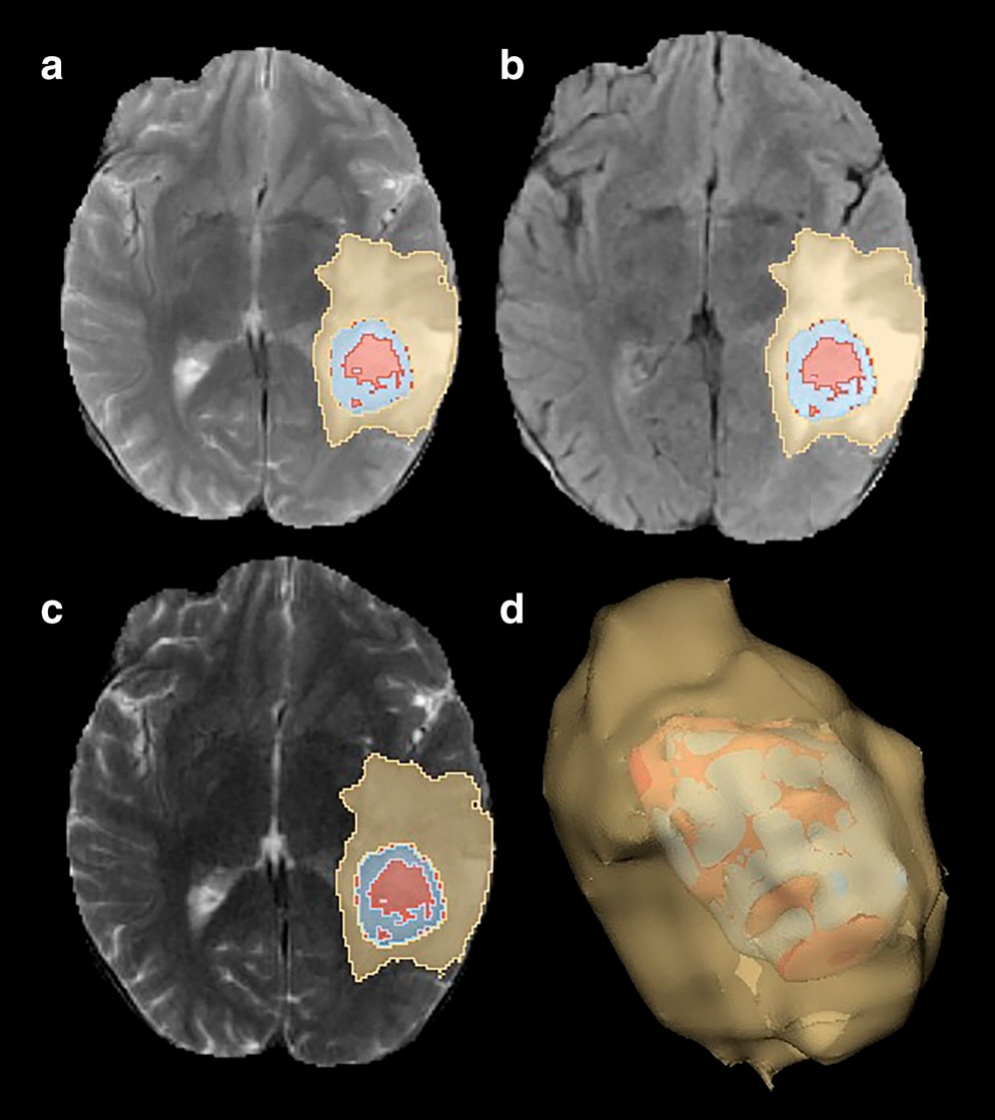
IDH wild Grade 4 Glioblastoma in the left cerebral hemisphere. (a) T2‐weighted images, (b) FLAIR‐weighted images, (c) T2‐FLAIR digital subtraction images, (d) 3‐dimensional volume rendering of the mass yellow color: perilesional edema area, blue color: contrast‐enhanced area, red: necrosis area.
